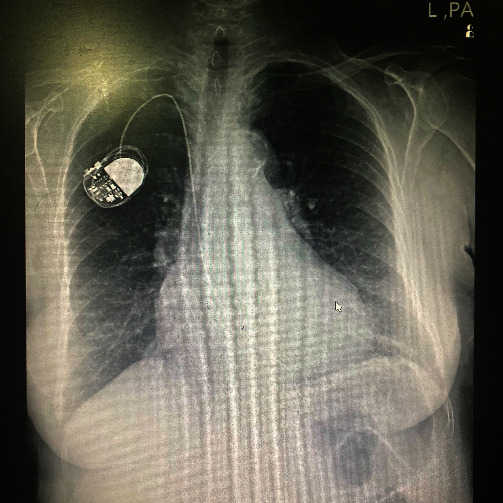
Upright chest X-ray without subdiaphragmatic free air.
